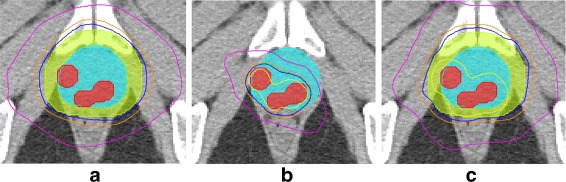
The treatment plans for EBRT shown on a single slice of T2w MRI, of a single patient - a
